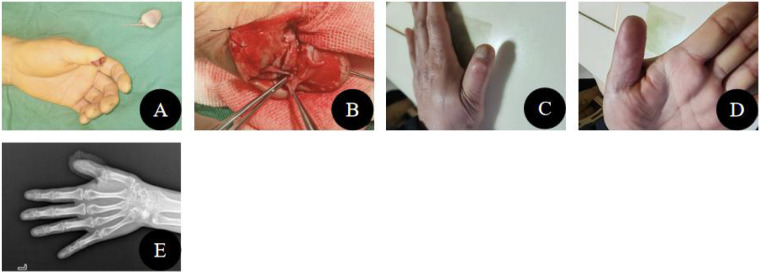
Case of venous reconstruction with proximal nail bed vein replacing the subcutaneous vein. (A) Amputation of the left thumb at the level of the nail root. (B) Anastomosis of a proximal nail bed vein with a dorsal subcutaneous vein. (C) Follow-up at 19 months postoperatively showing smooth nail growth. (D) Follow-up at 19 months postoperatively showing a well-preserved contour. (E) An x-ray taken two months after surgery showed that the fracture of the left thumb had healed well, and the internal fixation was removed.
